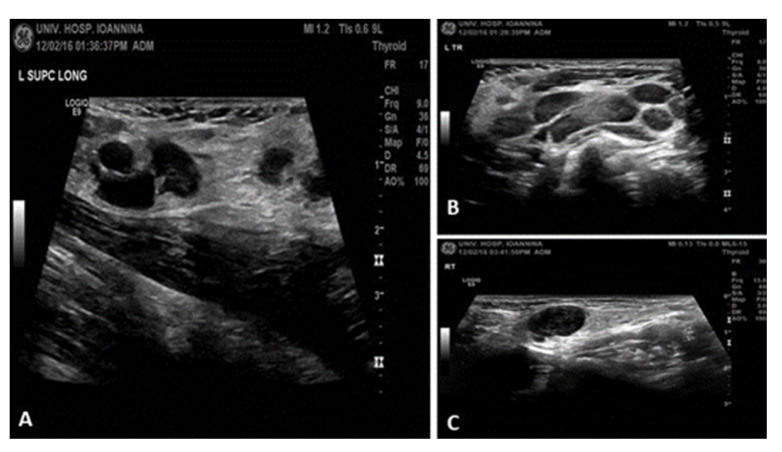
Ultrasound findings: (A) In the supraclavicular regions, multiple enlarged lymph nodes are also revealed that locally converge. Some of them appear hypoechoic and rounded; the largest is on the left with a maximum diameter of ~1.3 cm. (B,C) In the cervical regions and within the parotid glands, more commonly on the left, multiple enlarged lymph nodes of maximum diameter up to 2 cm are observed, which in some areas converge with an oval morphology and normal architecture.
